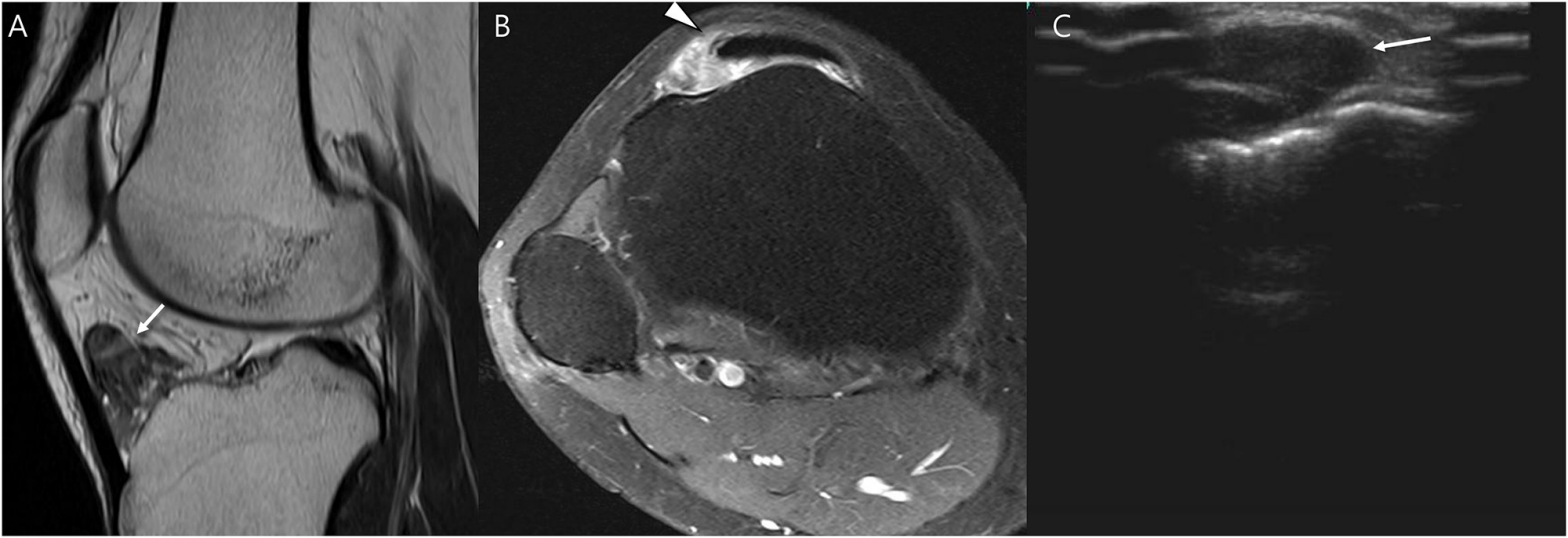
A 19-year old woman with pathology-proven intraarticular L-TSGCT of knee. (A and B) Preoperative sagittal and axial T2-weighted images show soft tissue mass located in the Hoffa’s fat pad with circumscribed margin containing granular internal hypointensity without visible peripheral hypointensity (arrow). Note that the patellar tendon is involved by tumor (arrowhead). (C) Follow-up ultrasound after 13 months show recurrent mass (arrow).
